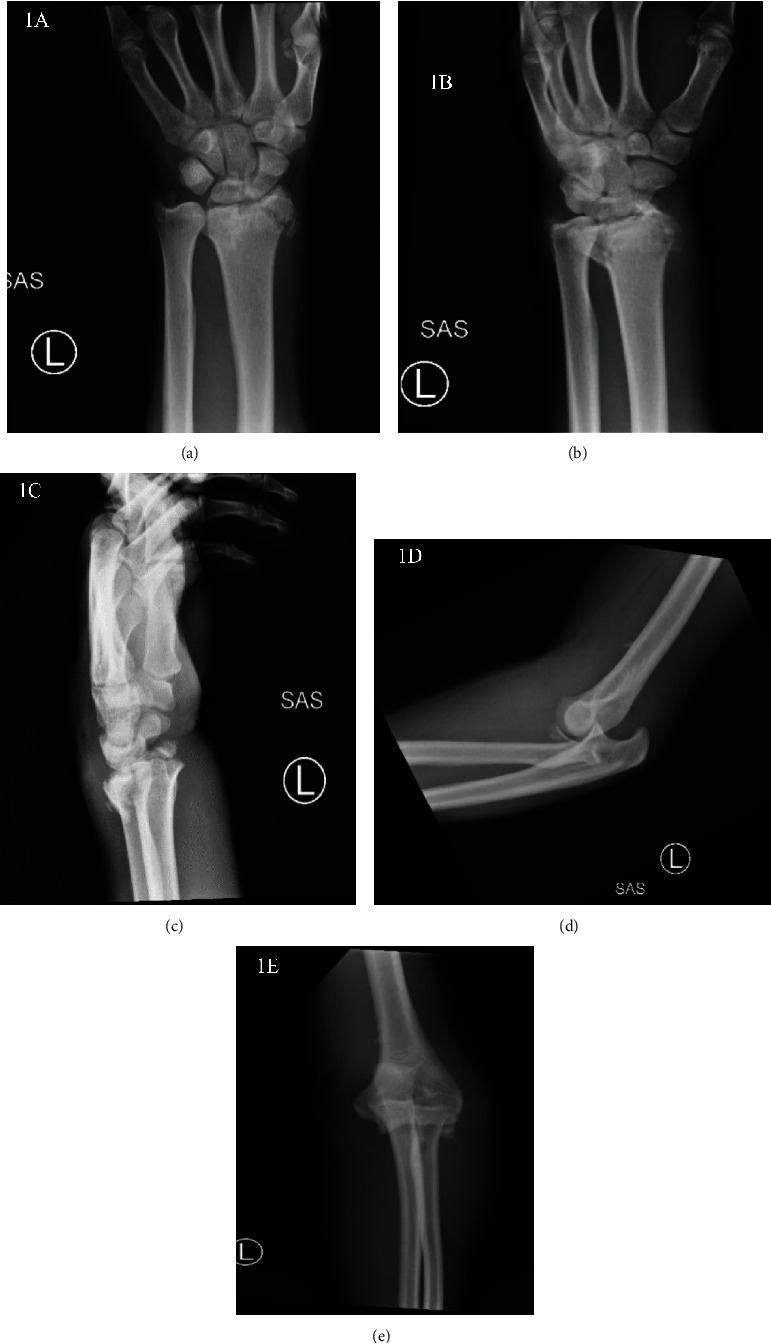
Left wrist PA (a), oblique (b), and lateral (c) injury radiographs along with left elbow lateral (d) and AP (e) injury radiographs. Left elbow posterior dislocation with fracture fragment along the anterolateral aspect of the elbow. Comminuted intraarticular left distal radius fracture.
